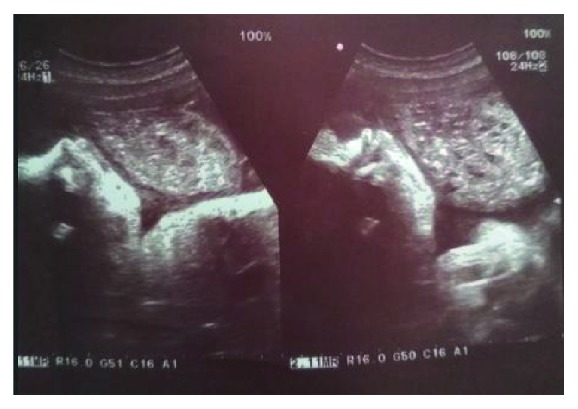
Ultrasound showing a heterogeneous cystic lesion anteriorly and a normal fetus.
